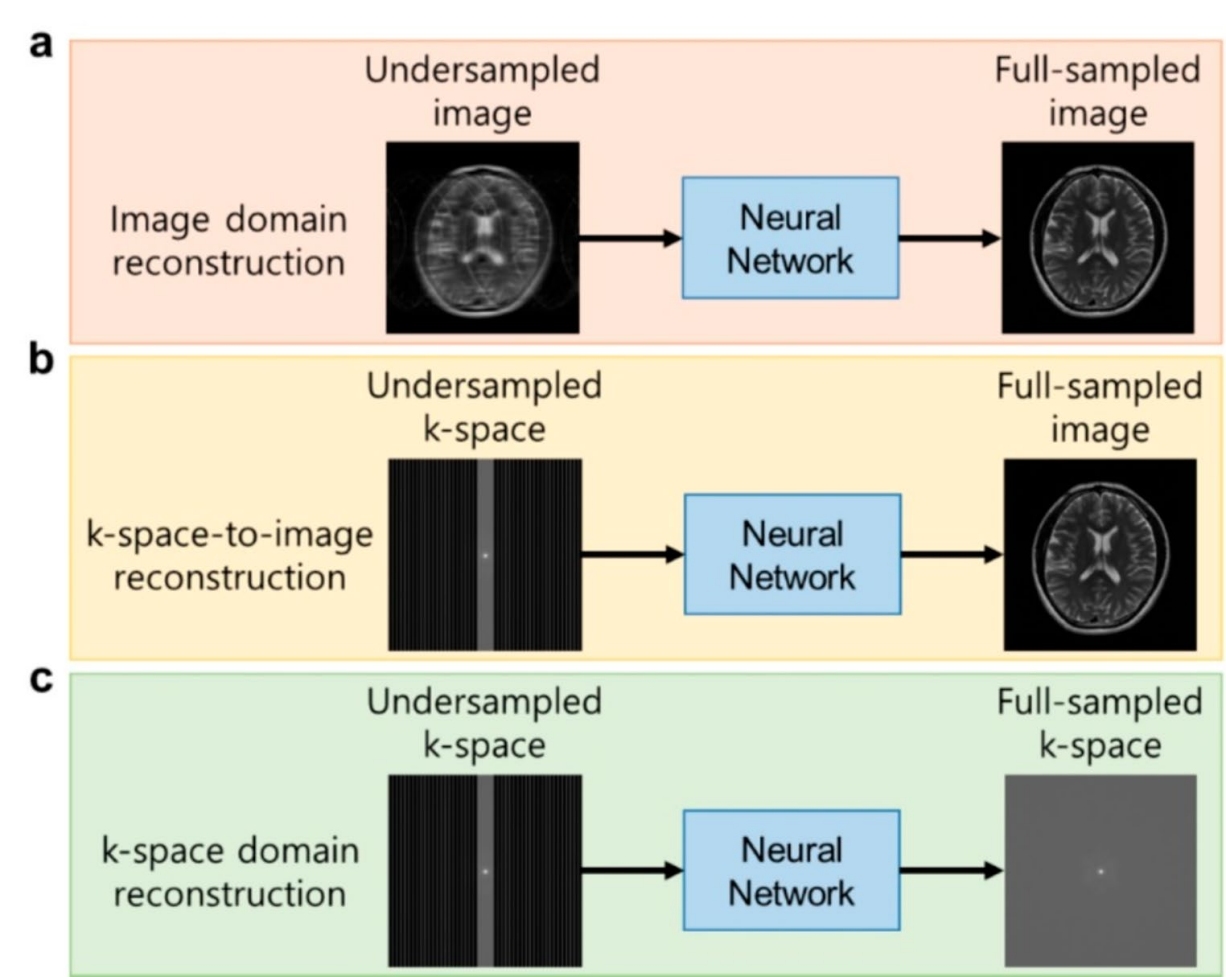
Various approaches for data-driven end-to-end learning for accelerated MRI reconstruction. a image domain reconstruction, where both the input and the output are in image domain. b k-space-to-image reconstruction, where the input is in k-space domain and the output is in image domain. c k-space domain reconstruction, where both the input and the output are in k-space domain
